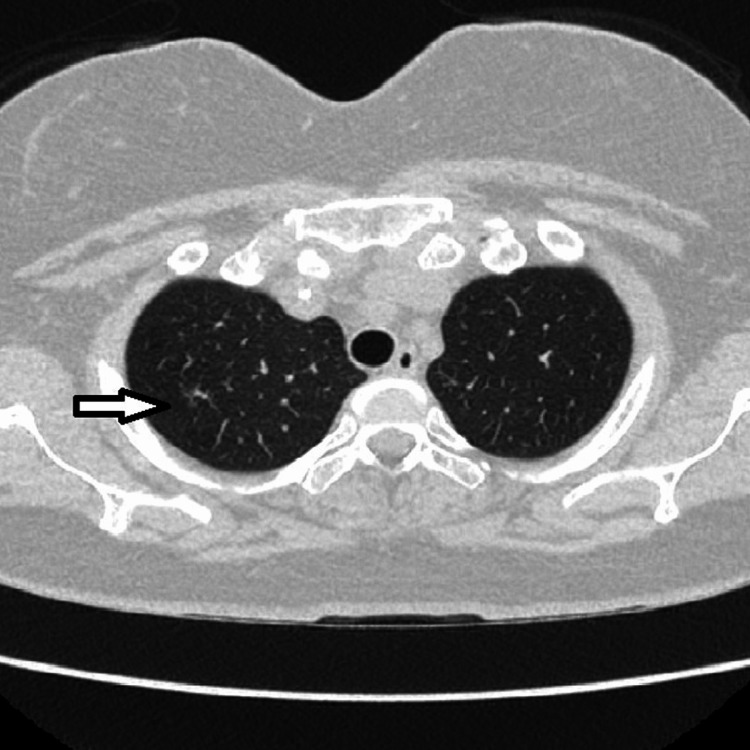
CT of the chest. Lung window. Lung upper lobes. Right upper lobe tree in bud opacities (arrow).
